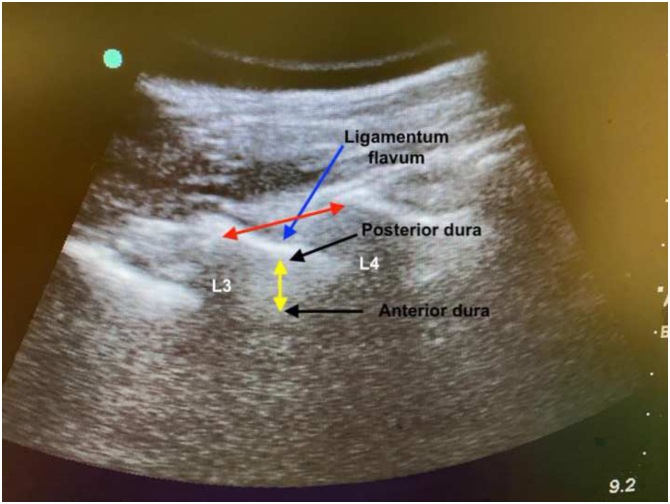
Paramedian longitudinal oblique ultrasonographic view of the lumbar spine at the level of the lamina showing the L3...4 and L4...5 interlaminar spaces. Red arrow: intervertebral distance; Yellow arrow: intrathecal space; L3: Lamina of L3 vertebrae; L4: Lamina of L4 vertebrae.
